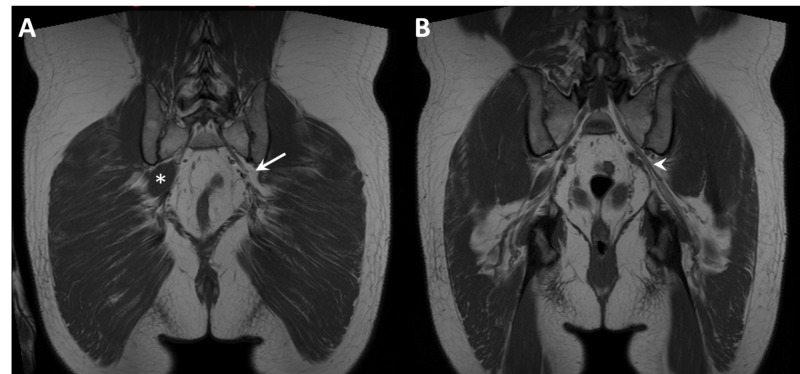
Magnetic resonance evaluation of the piriformis muscle. T1 weighted coronal magnetic resonance image slices show agenesis of the piriformis muscle on the left (A, arrow) and the normal right piriformis muscle (asterisk). The left sciatic nerve is highlighted (B, arrowhead).
